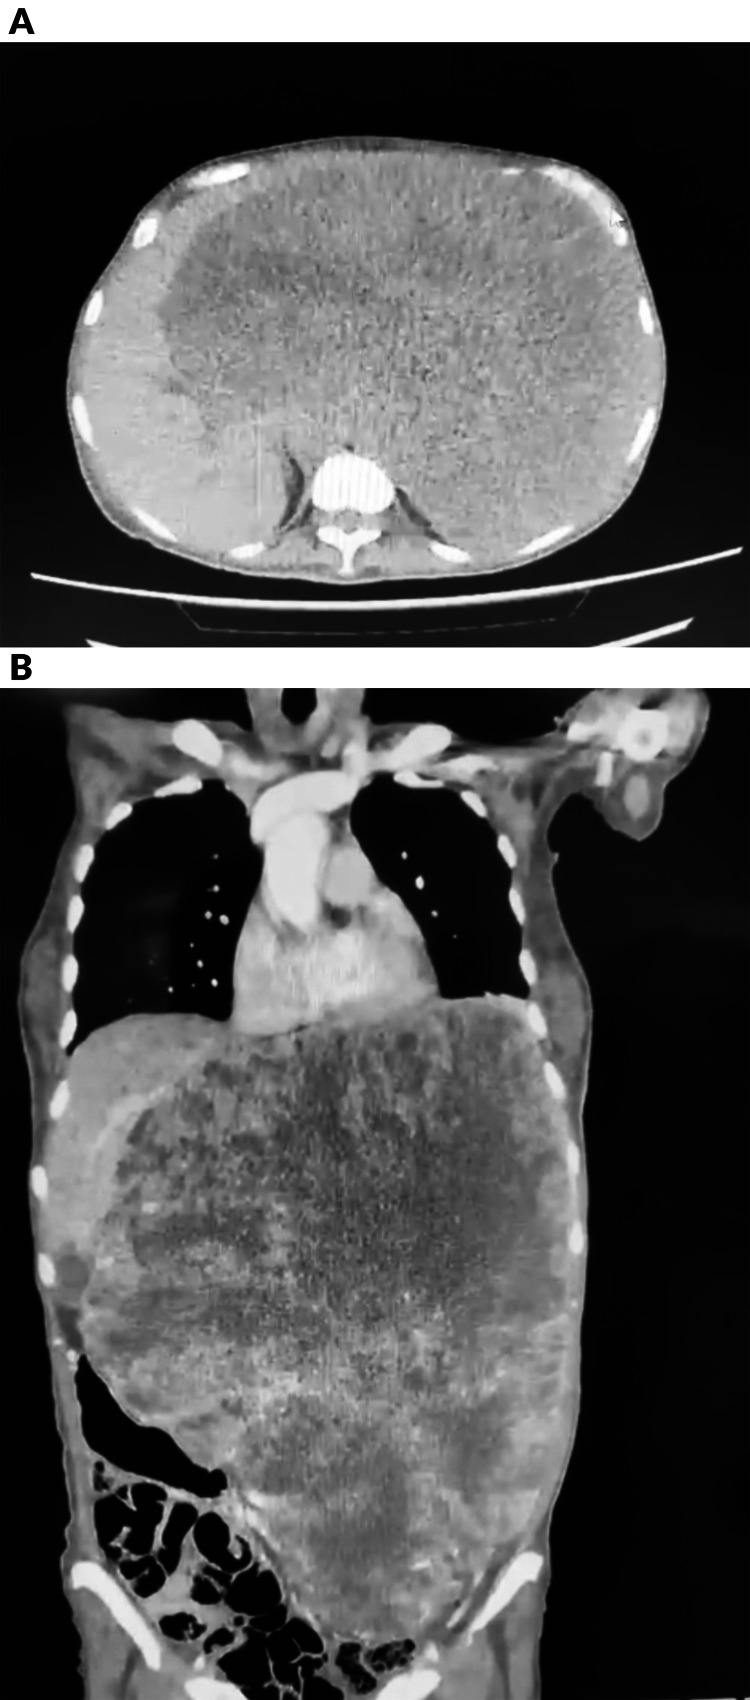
A. Axial view of the abdominal CT scan where an irregularly shaped tumor is observed, not delimited on the edges of the stomach. B. Coronal view of abdominal CT scan where it is observed that the tumor exerts a significant mass effect on adjacent structures, displacing them contralaterally, preserving the interface. The tumor measures 248x154x345 mm.
